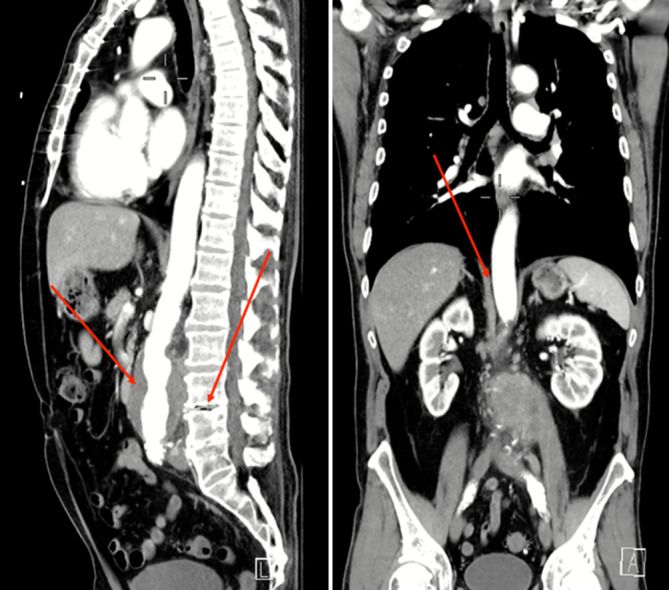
Ausgangsbefund (CT, sagittale und koronare Rekonstruktionen) des infrarenalen MBAA und angrenzender entzündlicher Prozesse im M. psoas links sowie dorsalseitig im Bandscheibenfach L3/L4. Die *roten Pfeile* zeigen auf das Aortenaneurysma, Lufteinschlüsse im Bandscheibenfach L3/L4 und den Psoasabszess (Quelle: Klinik für Radiologie und Nuklearmedizin, Universitätsklinikum Magdeburg A. ö. R.)
